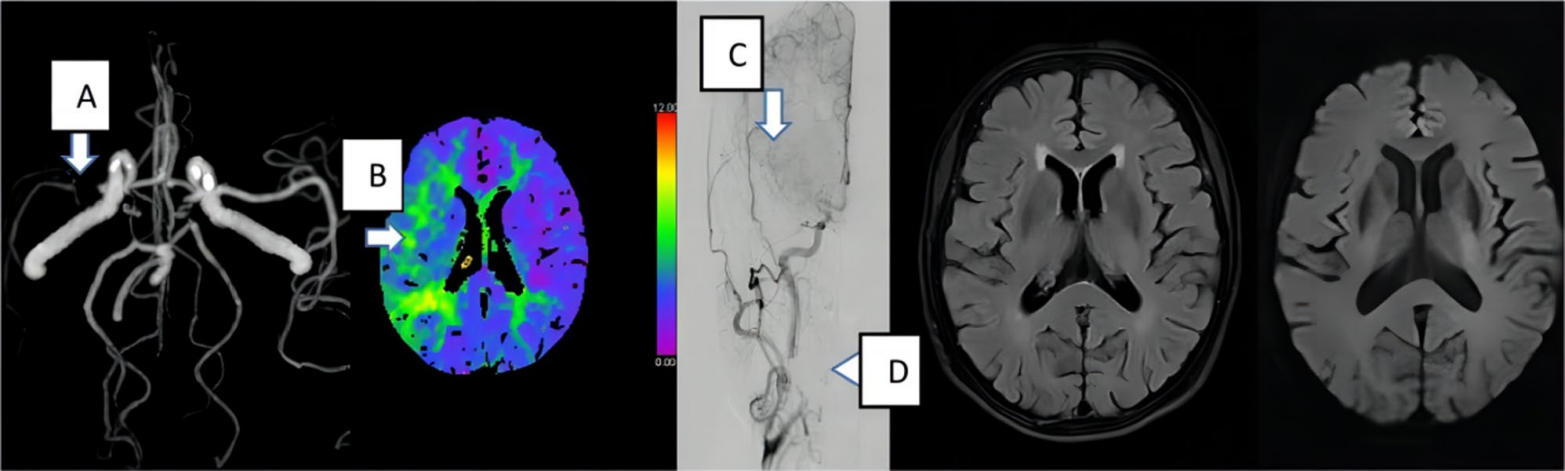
CTA images of a patient, suggesting severe stenosis of the right MCA (arrow A), and CTP images suggesting a large area of prolonged Tmax in the right MCA perfusion region (arrow B). The DSA image indicates occlusion of the right MCA (arrow C). The area indicated by triangle C was the location of the patient’s denture. There was no previous brain infarction apparent on the MRI T2FLAIR image, or acute ischemic stroke on the MRI DWI image. The interpretation times of the CTA images of the patient were 73 s for R1 and 81 s for R2. After the addition of CTP maps, the interpreting times were 71 s for R1 and 78 s for R2
